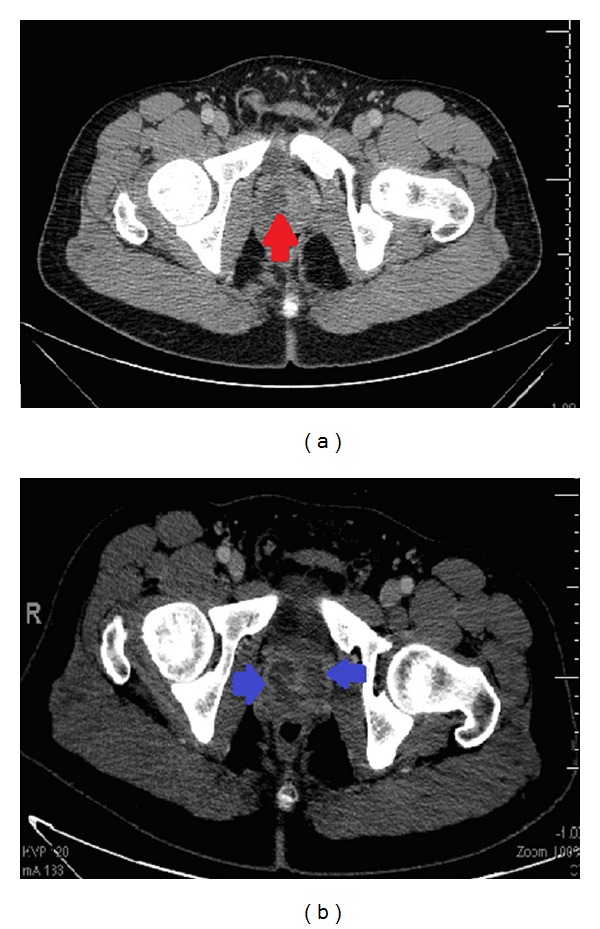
(a) Initial CT scan (left) showing 2.3 cm prostate abscess in the right gland (red arrow). (b) Repeat CT scan (right) 1 week later showed larger and multiple cystic fluid collections in the prostate gland (blue arrow).
